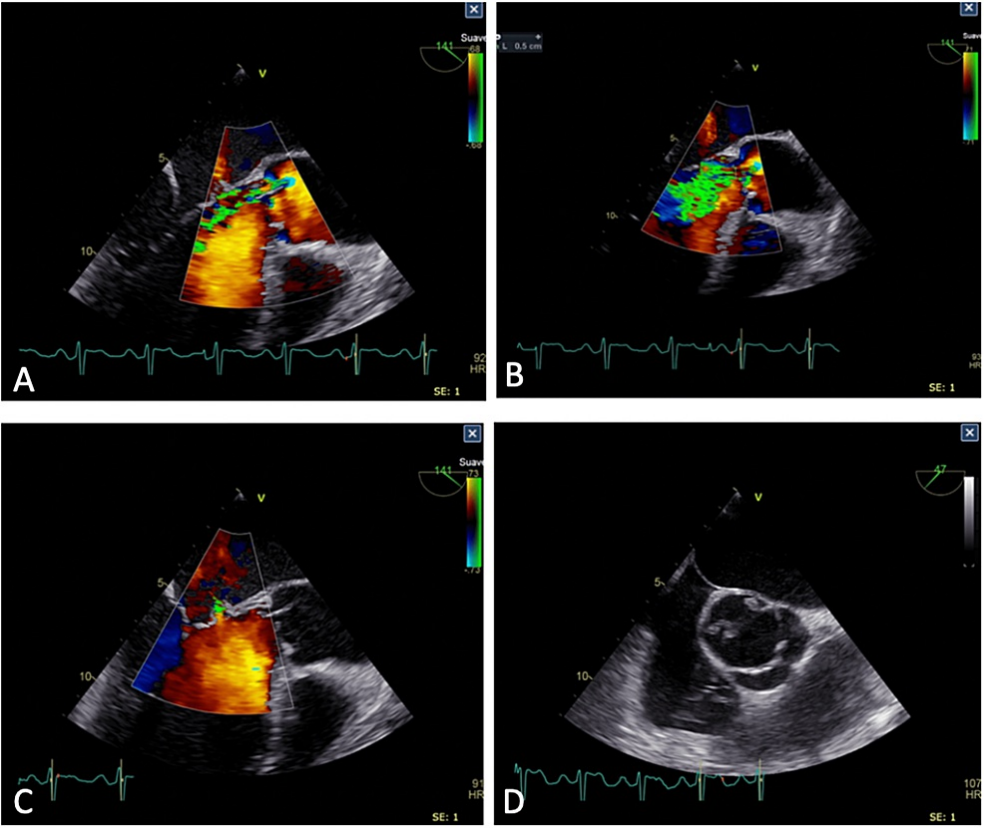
Transthoracic echocardiogram revealing abnormalities consistent with infective endocarditis. 2A, 2B: Evidence of marked aortic insufficiency with two jets (one eccentrical and one centrical). 2C: Evident perforation as a consequence of the abscess, which resulted in an eccentric jet. 2D: 2D echocardiogram showing vegetation on the bicuspid aortic valve
